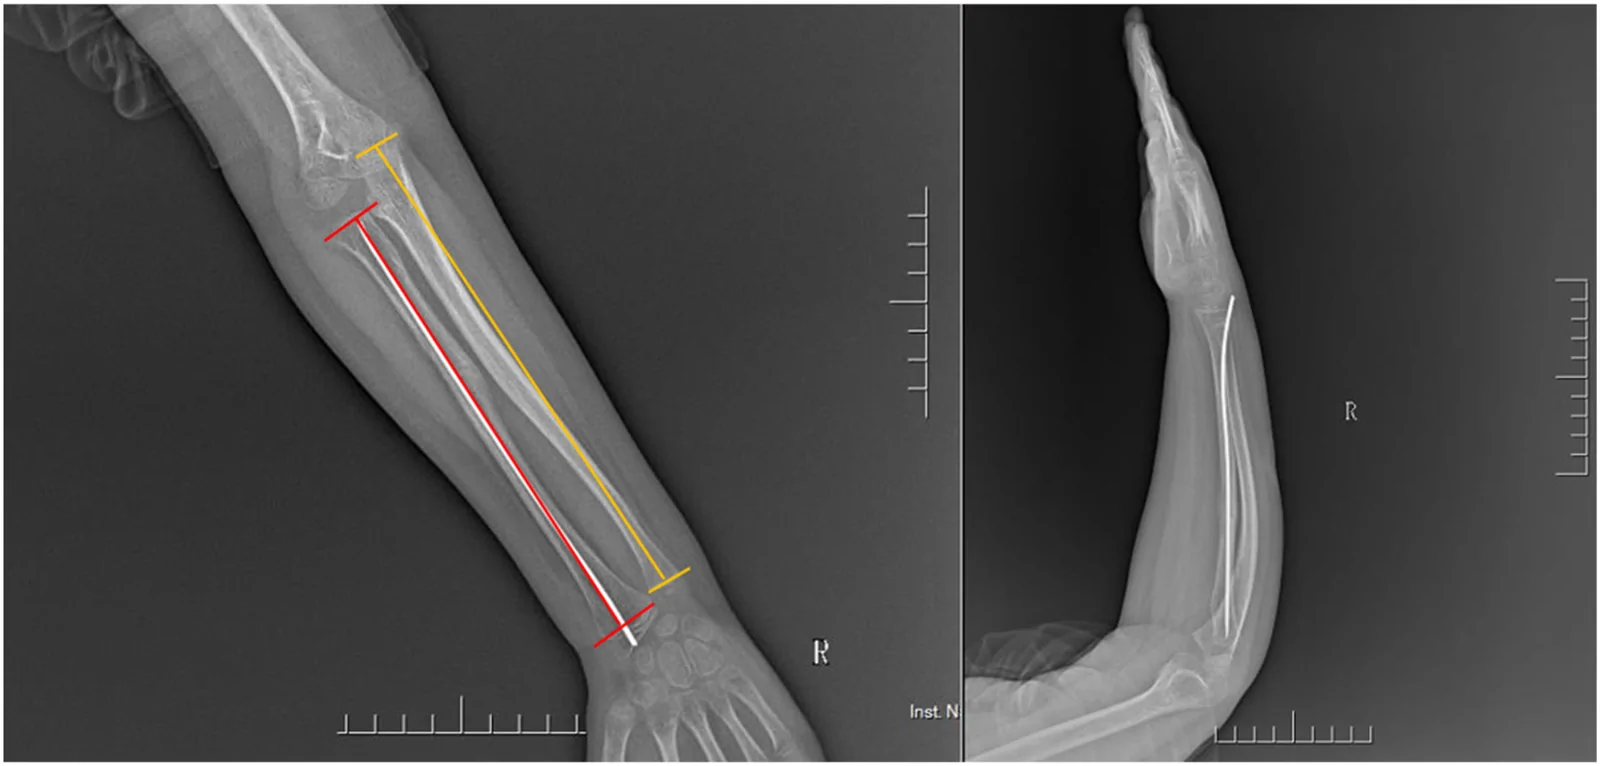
Two-month postoperative follow-up radiograph demonstrating solid radial fracture union; measured inner epiphyseal ulna-radius length ratio = 1.087.
